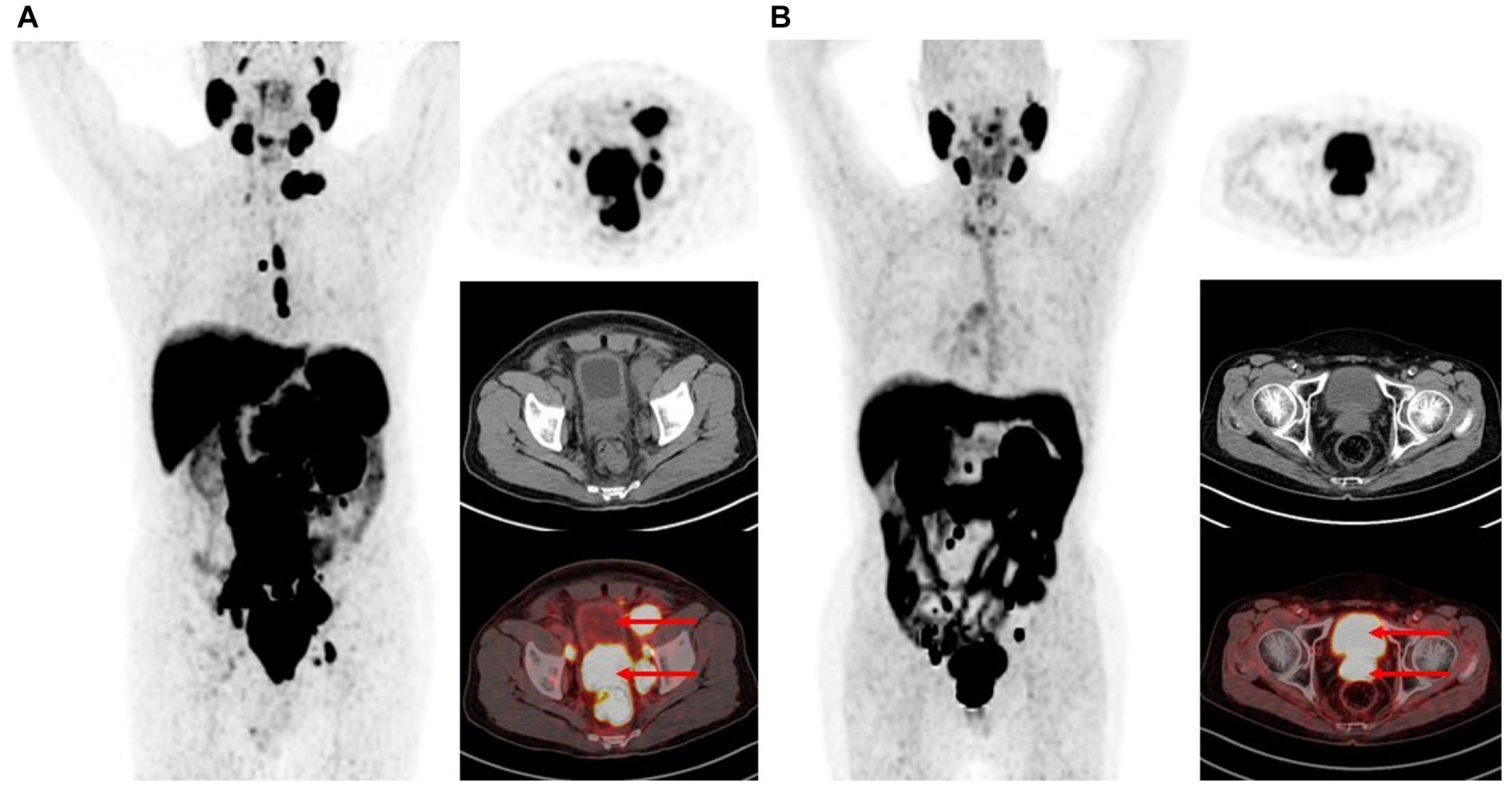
Maximum intensity projections and PET, CT, fusion image of the urinary bladder uptake on ^18^F-PSMA-1007 PET/CT. (A) Bladder SUV_max_ is 1.8. (B) Bladder SUV_max_ is 17.0.
